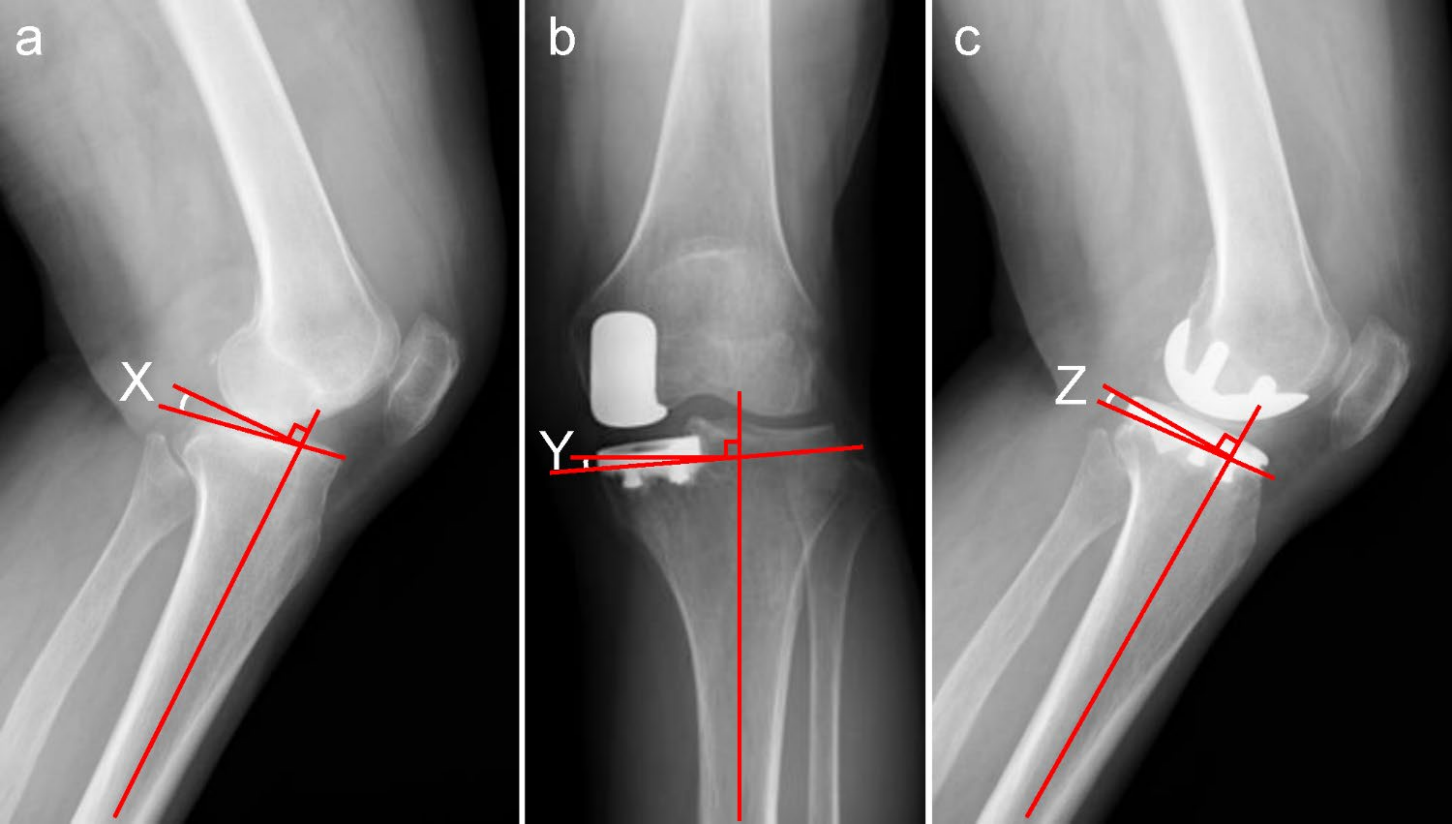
Measurement methods in radiographic assessments. a Preoperative PTS (angle X) was defined as the angle between the line perpendicular to the tibial bone axis and the medial tibial plateau in the lateral radiograph of the knee joint. b Postoperative TI (angle Y) was defined as the angle between a line perpendicular to the tibial bone axis and the inferior surface of the tibial component in the anteroposterior radiograph of the knee joint. c Postoperative PTS (angle Z) was defined as the angle between the line perpendicular to the tibial bone axis and the inferior surface of the tibial component in the lateral radiograph of the knee joint. *PTS* posterior tibial slope, *TI* tibial inclination
